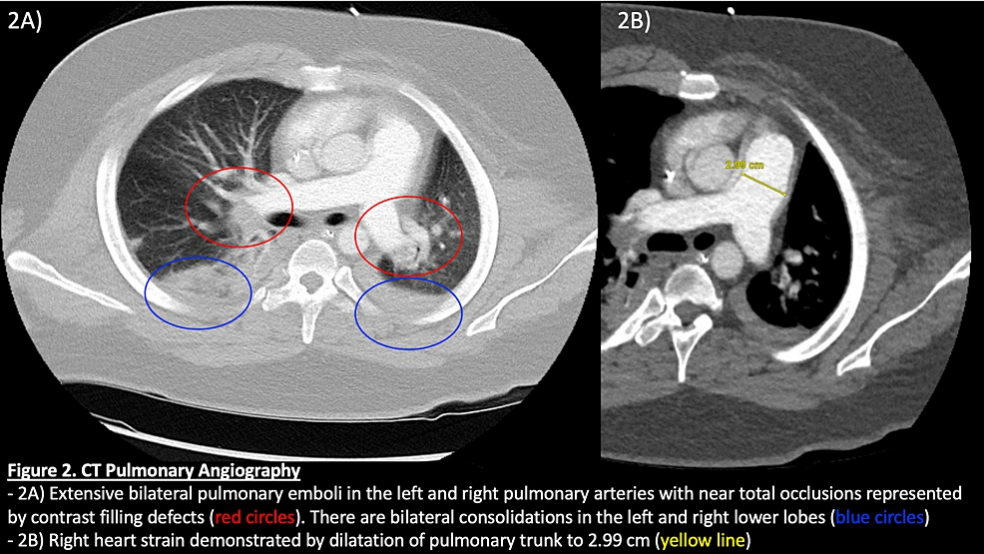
CT Pulmonary Angiography of Bilateral Pulmonary Emboli - 2A) Extensive bilateral pulmonary emboli in the left and right pulmonary arteries with near total occlusions represented by contrast filling defects (red circles). Bilateral consolidations can also be seen in the left and right lower lobes (blue circles). - 2B) Evidence of right-sided heart strain is demonstrated by dilatation of the pulmonary trunk to 2.99 cm (yellow line).
